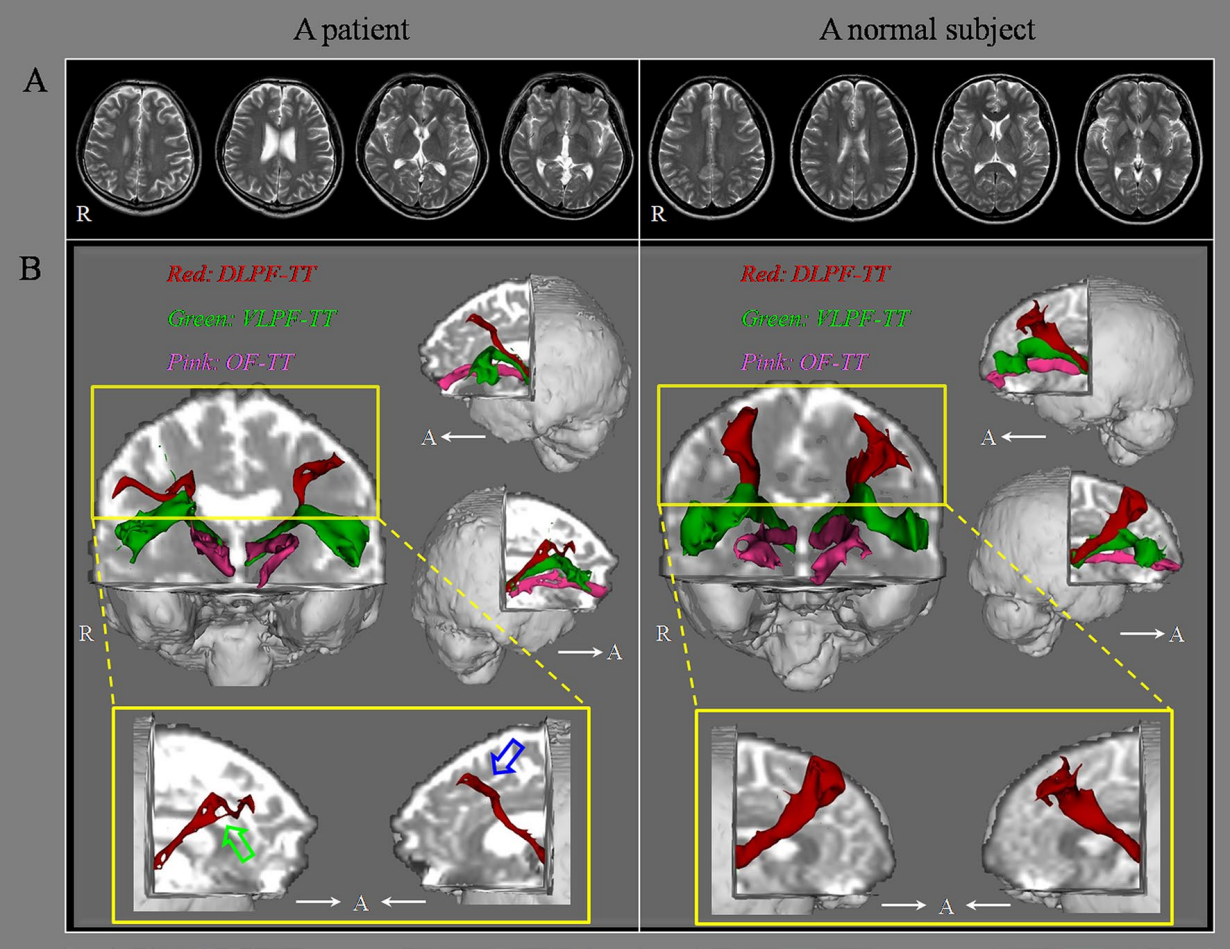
(A) T2-weighted brain magnetic resonance images showing no abnormalities in a representative patient (35-year-old male) and a normal subject (37-year-old male). (B) Results for the three prefronto-thalamic tract (PF-TT) regions on diffusion tensor tractography: DLPF-TT—dorsolateral prefronto-thalamic tract (red), VLPF-TT—ventrolateral prefronto-thalamic tract (green), and OF-TT—orbitofronto-thalamic tract (pink). The DLPF-TT in a patient shows partial tearing (green arrow) in the right hemisphere and narrowing (blue arrow) in the left hemisphere compared that that in a normal subject.
